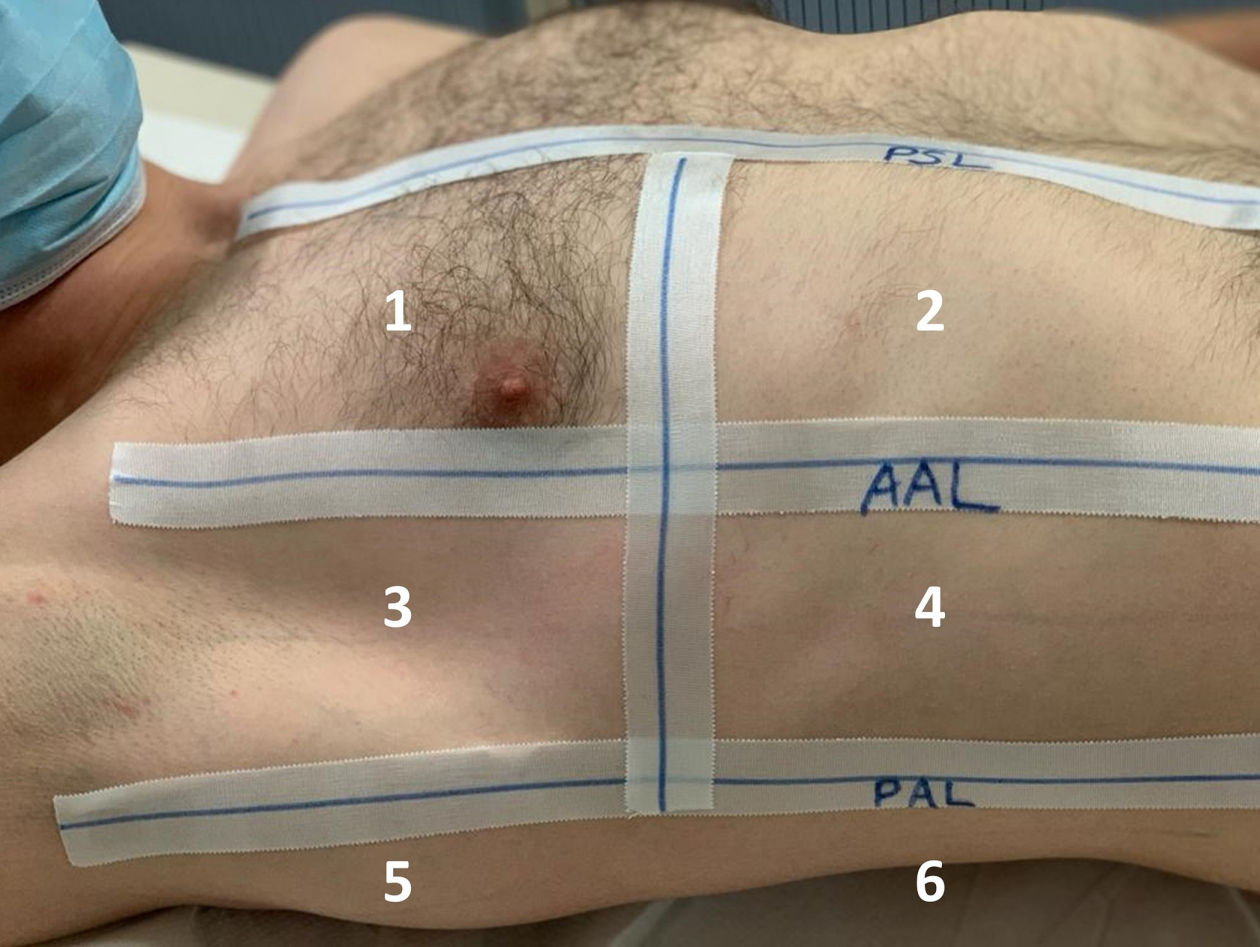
Lung areas for the right lung, PSL = parasternal line, AAL/PAL = anterior/posterior axillary line.
